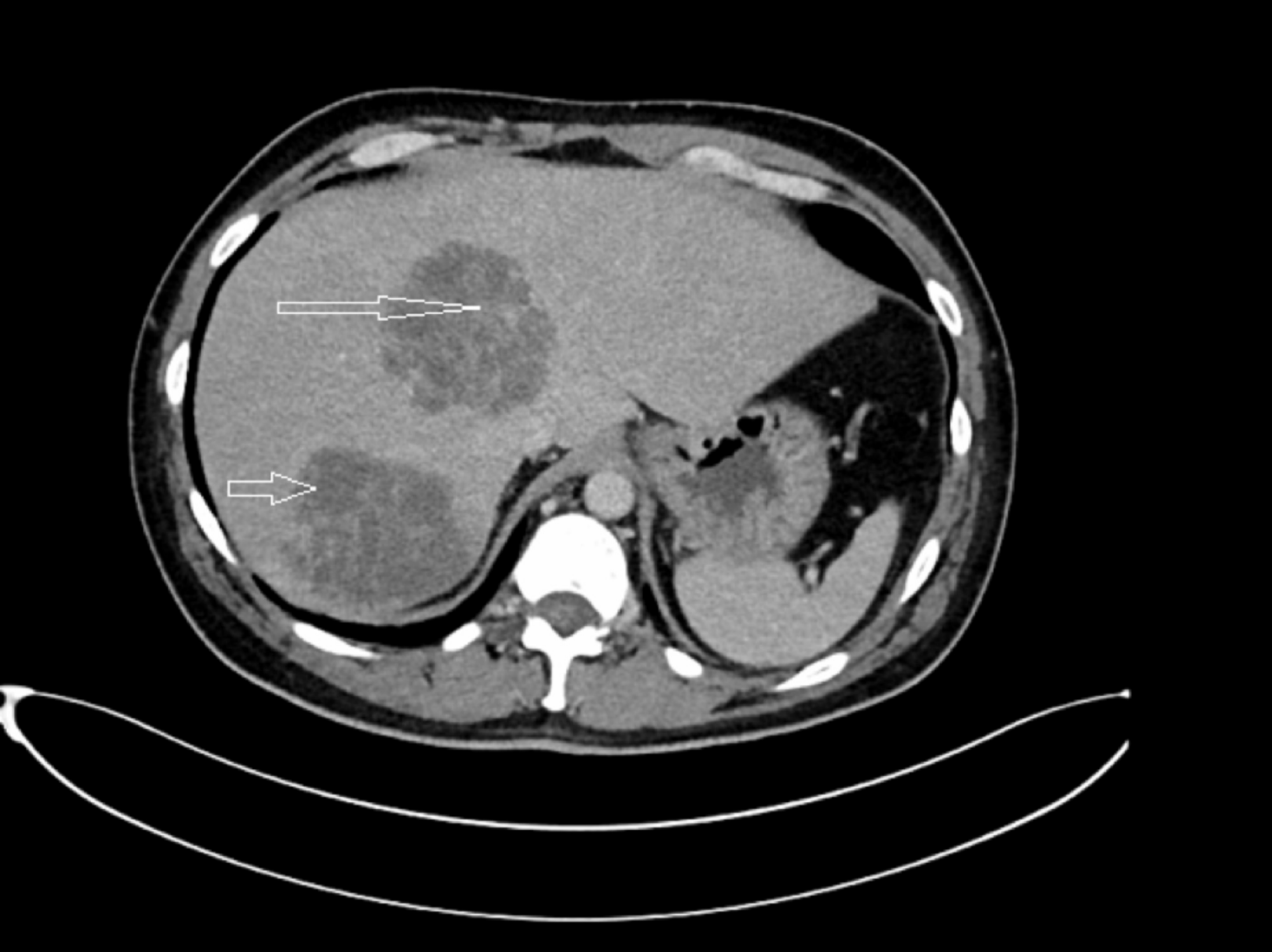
CT abdomen done in October 2019. Depicting two large, multiloculated abscesses in right lobe of liver, measuring each 7-8 cm in size.
